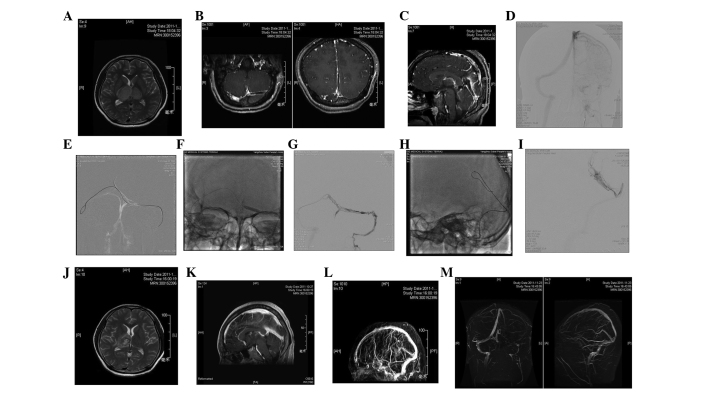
Catheter-directed thrombolysis in the straight sinus. (A) Head MRI T2 weighted image showed that the bilateral thalami were infarcted and the right side was more severe; (B) thrombus in the straight sinus as shown by enhanced MRI; (C) thrombus in the left transverse sinus and superior sagittal sinus as shown by enhanced MRI; (D) DSA showed that the left transverse sinus and straight sinus did not develop; (E) guidewire entered the transverse sinus during surgery; (F) sacculus expanded during surgery; (G) left transverse sinus was open during surgery; (H) microcatheter was placed in the straight sinus; (I) microcatheter angiography revealed that the straight sinus developed following thrombolysis; (J) 2 weeks after surgery, review of the T2 weighted image showed that disease extent of the thalamus had reduced; (K) enhanced imaging showed that the superior sagittal sinus and straight sinus were open; (L) MRV showed that the superior sagittal sinus and straight sinus were open; (M) 6 weeks after surgery, MRV showed that the straight sinus was open but the left transverse sinus was closed. CT, computed tomography; MRI, magnetic resonance imaging; DSA, digital subtraction angiography; MRV, magnetic resonance venography.
